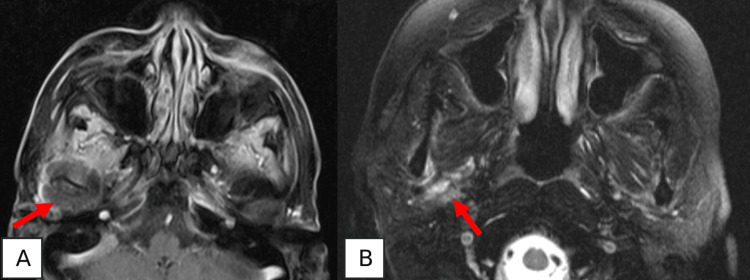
Magnetic resonance imaging four months after treatment No evidence of organized abscesses. (A) Axial T1 FS post-gadolinium image showing synovial thickening with slight enhancement (red arrow), suggestive of synovitis with some fibrosis. (B) Axial T2 FS image showing slight condylar edema (red arrow) FS: fat-saturated
